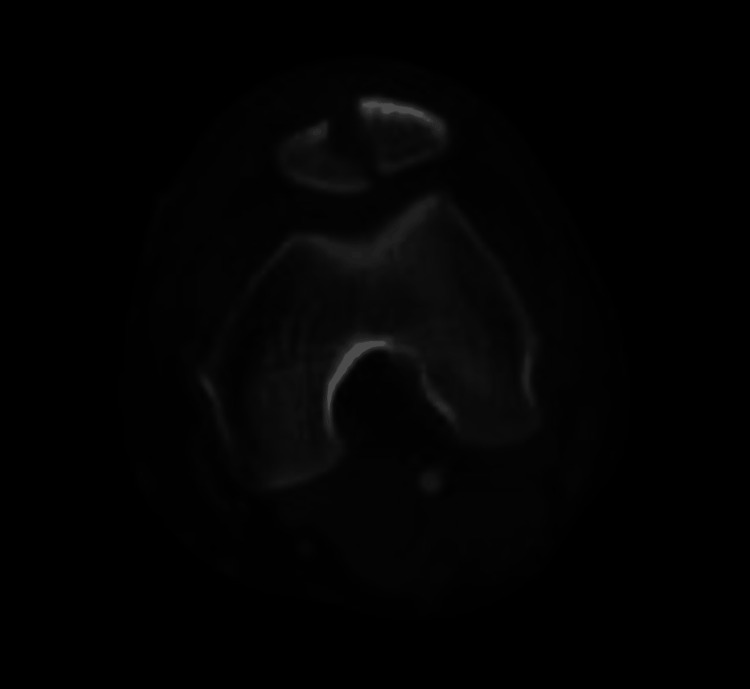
CT scan demonstrating a displaced vertical medial sagittal patellar fracture.
